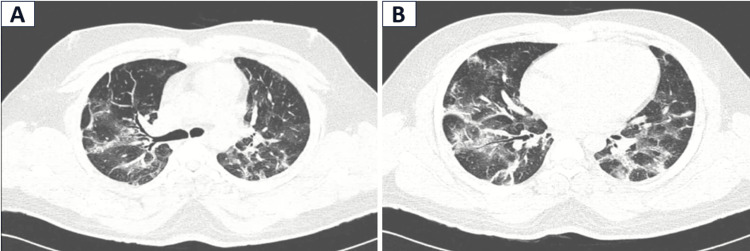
(A and B): HRCT Thorax showing ground glass opacities, airspace consolidation with air bronchograms, and interlobar septal thickening
